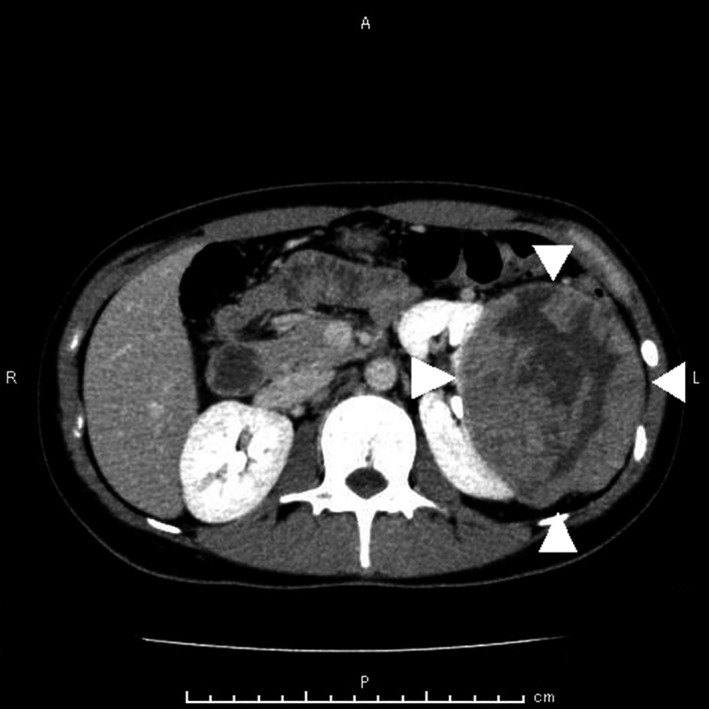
Contrast‐enhanced computed tomography showed a 13 × 10‐centimeter, multiloculated mass with septations on the left kidney (*arrowheads*)
